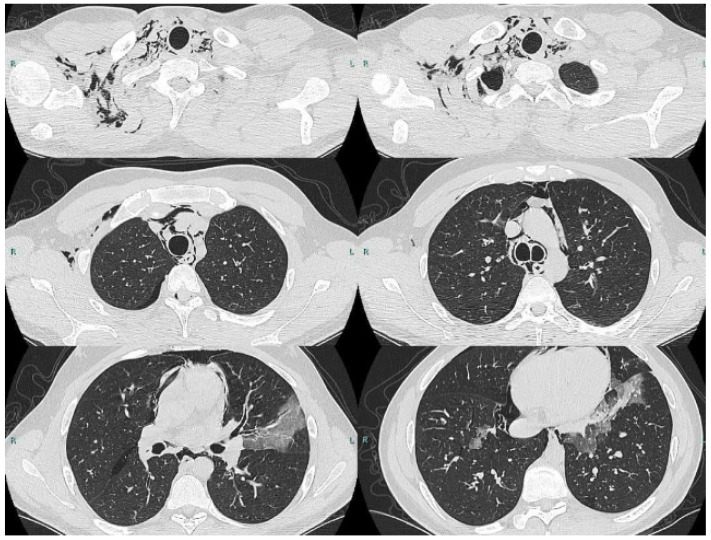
Chest CT scan at admission.
